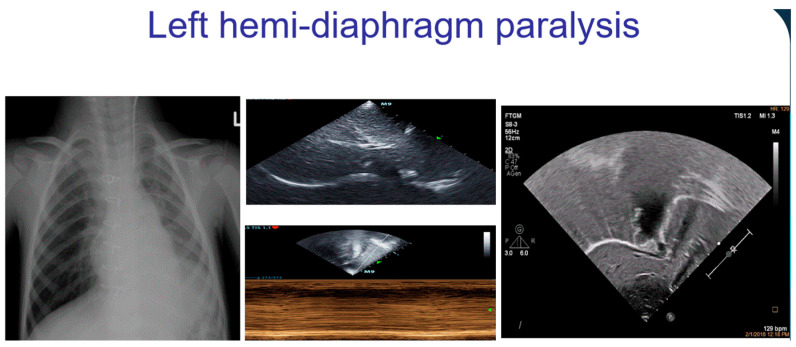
And Video S2: A left hemidiaphragm paralysis can be visualized by chest X-ray (left side) and confirmed by echographic analysis of diaphragm by subcostal view. In the middle, a paradoxical motion of the diaphragm can be appreciated in M-mode, and on the right image, the left hemidiaphragm lifted can be appreciated (Video S2).
